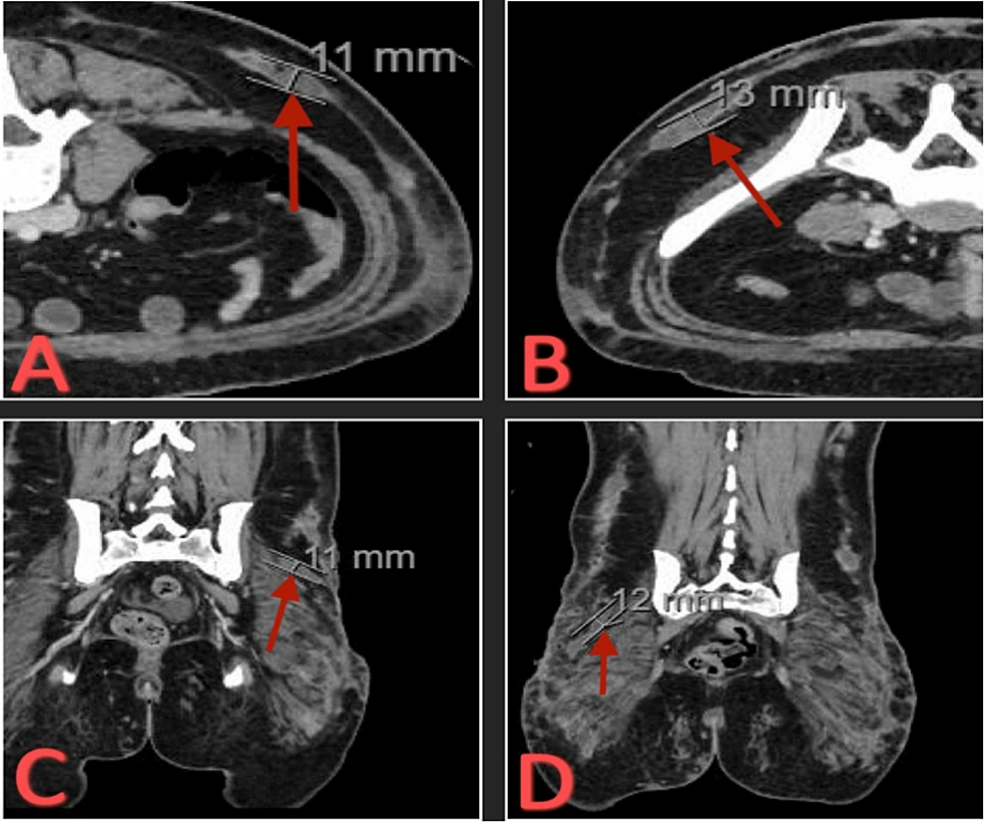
CT abdomen and pelvis with contrast. (A) Transverse view of 11 mm subcutaneous soft tissue micro-abscess. (B) Transverse view of 13 mm subcutaneous soft tissue micro-abscess. (C) Coronal view of 11 mm subcutaneous soft tissue micro-abscess. (D) Coronal view of 12 mm subcutaneous soft tissue micro-abscess.
